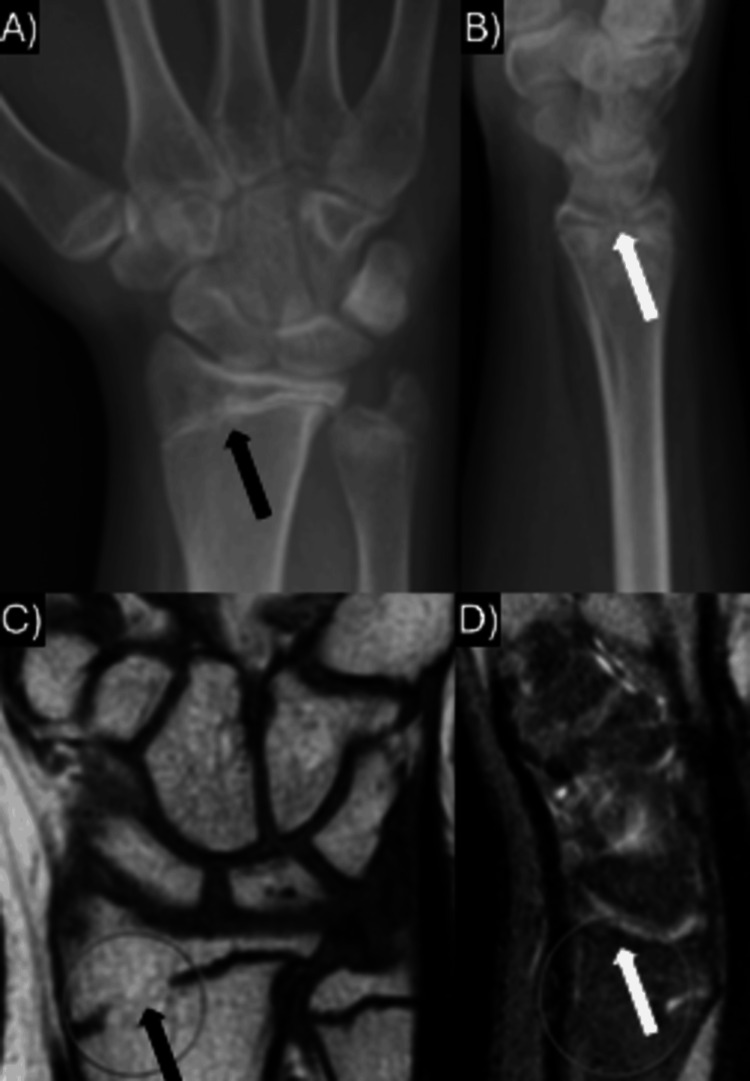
Plain radiographs and MRI of case 11 obtained at the 15-month follow-up showing decreased radial inclination and radially-based physeal bar formation. A) Case II's AP plain radiographs at the 15-month follow-up; B) Case II's lateral plain radiographs at the 15-month follow-up; C) Case II's coronal T1 MRI imaging at the 15-month follow-up; D) Case II's sagittal T1 MRI imaging at the 15-month follow-up. Black arrows in panes A and C demonstrate the physeal bar on AP radiographs and coronal MRI imaging, while white arrows in panes B and D demonstrate the physeal bar on lateral radiographs and sagittal MRI imaging. AP: anterior-posterior
